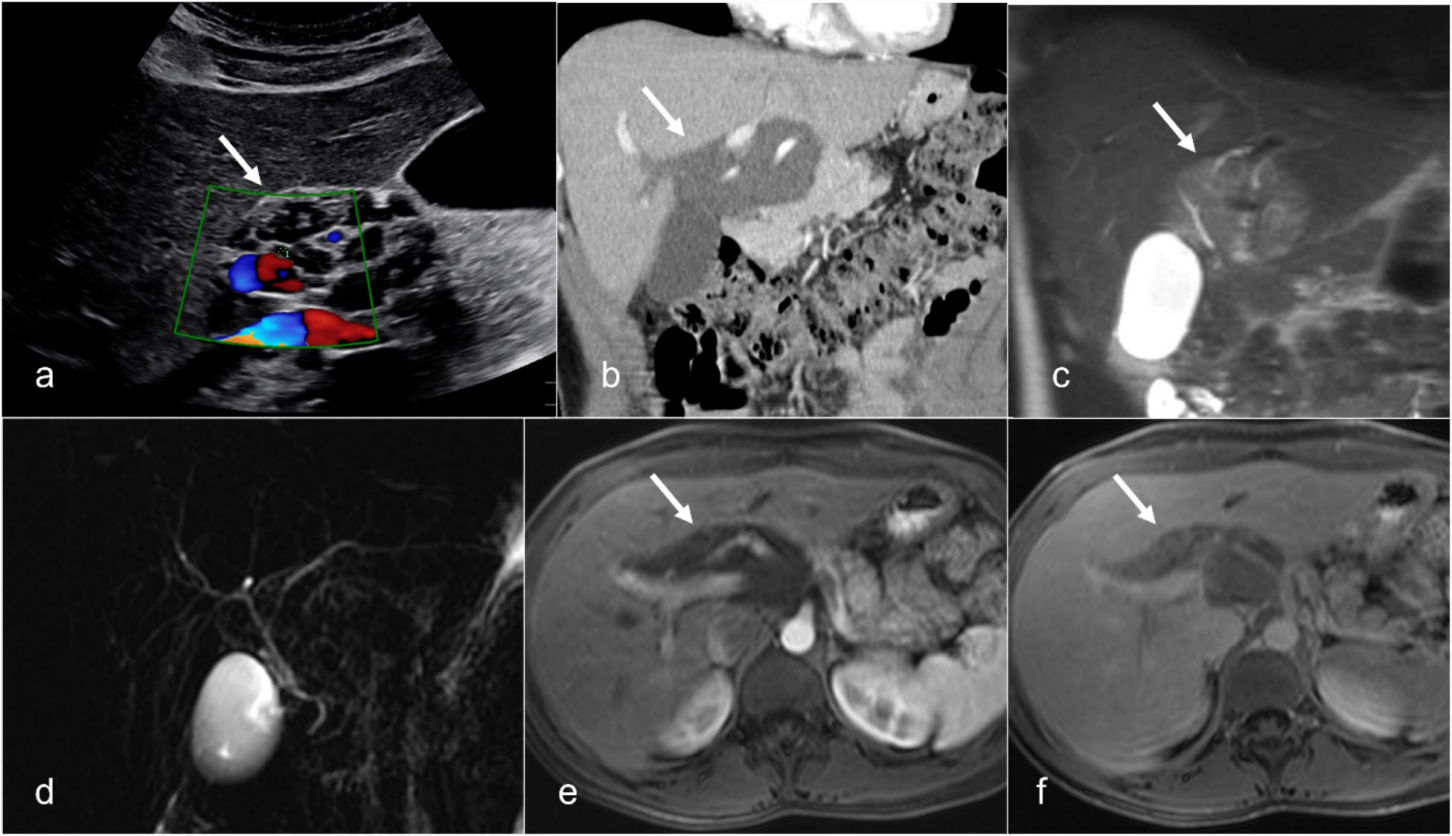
Neurofibromatosis in a 23-year-old patient with abdominal pain. Color Doppler US image (a) shows anechoic appearing cystic lesion with no blood flow. Coronal CT with contrast (b) demonstrates a poorly enhancing infiltrating lesion along the hepatic vasculature. Coronal MRI T2-weighted fat-saturated (c) images show T2-weighted hyperintense lesion (arrow) infiltrating along the bile ducts. MR cholangiopancreatography maximum intensity projection image (d) shows no bile duct dilatation. Postcontrast T1-weighted arterial (e) and delayed phase (f) shows delayed enhancement of the lesion (arrow). The lesion was biopsied to confirm the finding of a plexiform neurofibroma
